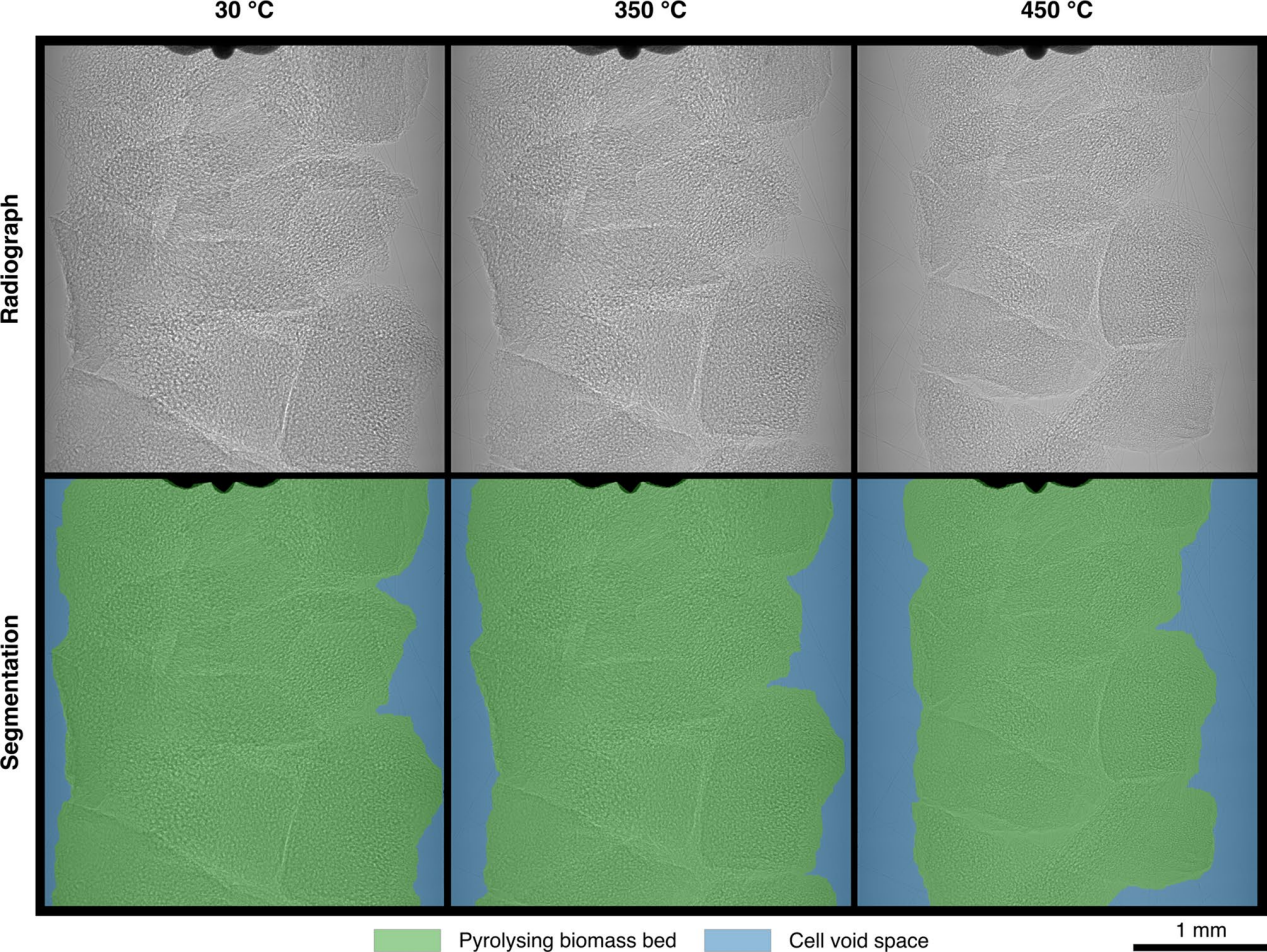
Radiographs and segmentations of a bed of water-soaked almond shells undergoing pyrolysis at a heating rate of 6 °C min^−1^. Radiographs were acquired using a pink synchrotron X-ray beam (weighted mean energy ~ 27 keV) with effective pixel size of the detector set as 1.625 μm with an exposure time of 0.05 s.
